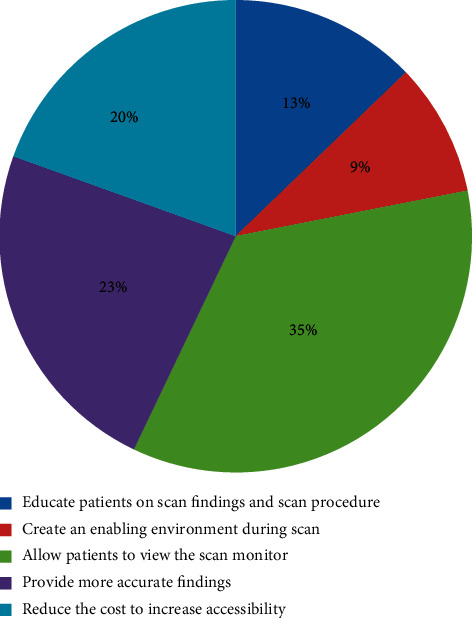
Participants' suggestions to doctors to make their experience better during their next ultrasound imaging.
